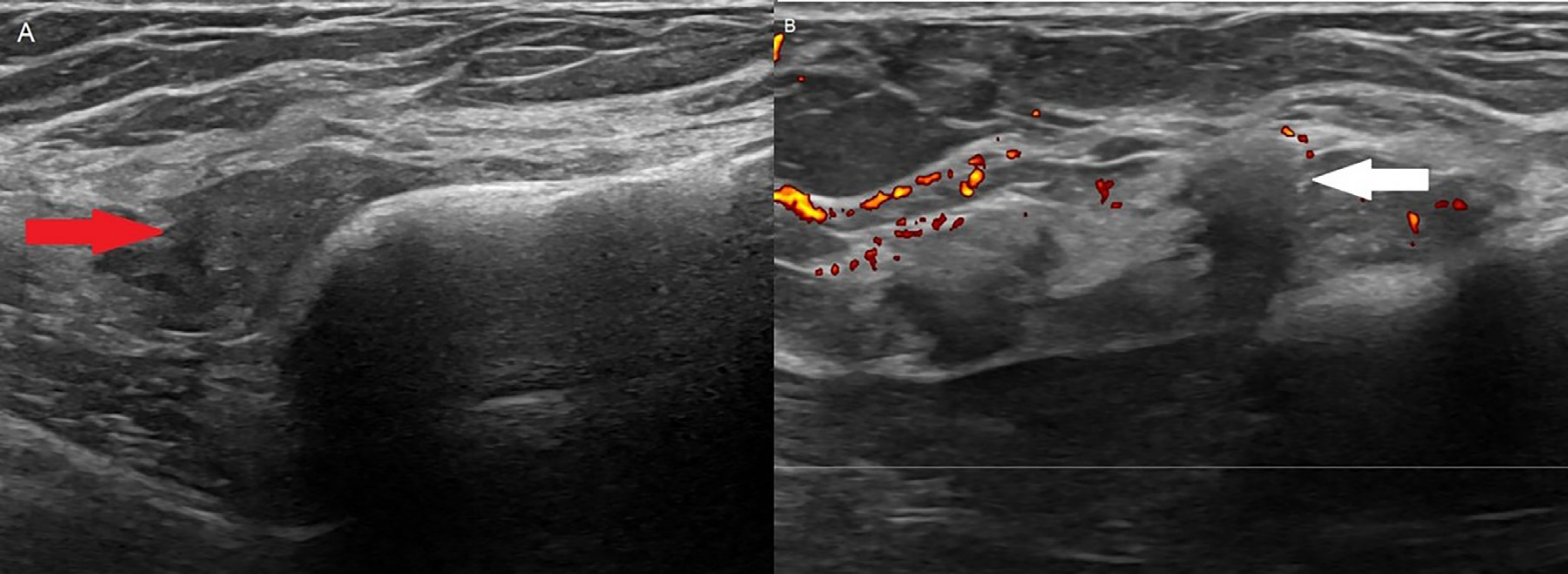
Grayscale ultrasound image (A) demonstrates an irregular, hypoechoic mass (red arrow) measuring approximately 2.2×0.8×1.9 cm along the implant capsule. Power doppler image (B) demonstrates the mass (white arrow) with no significant vascularity.
